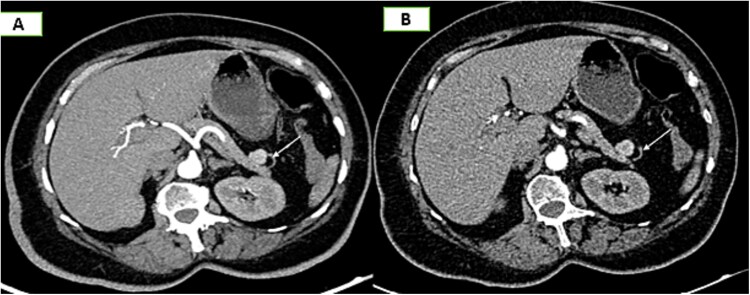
Axial sections of an abdominal CT scan in the arterial phase (A) and vascular reconstructions (B) demonstrate the vascularization of the accessory spleen through its pedicle.
